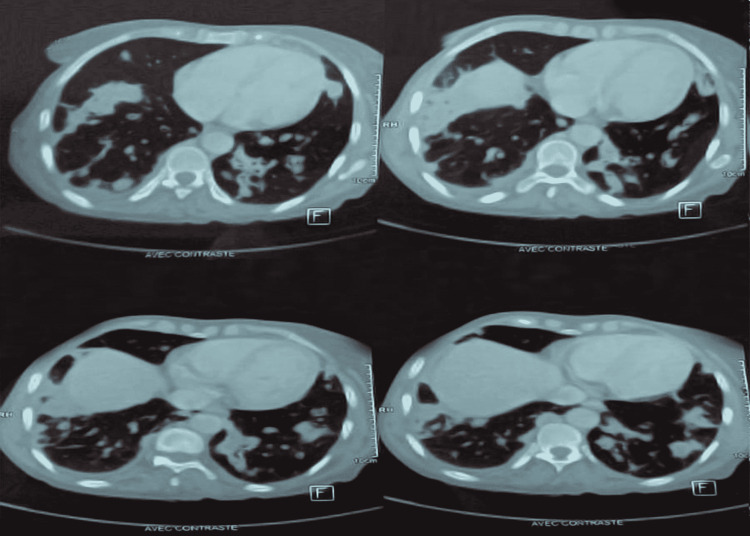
Chest computed tomography, parenchymal window: multiple foci of bilateral parenchymal condensation
